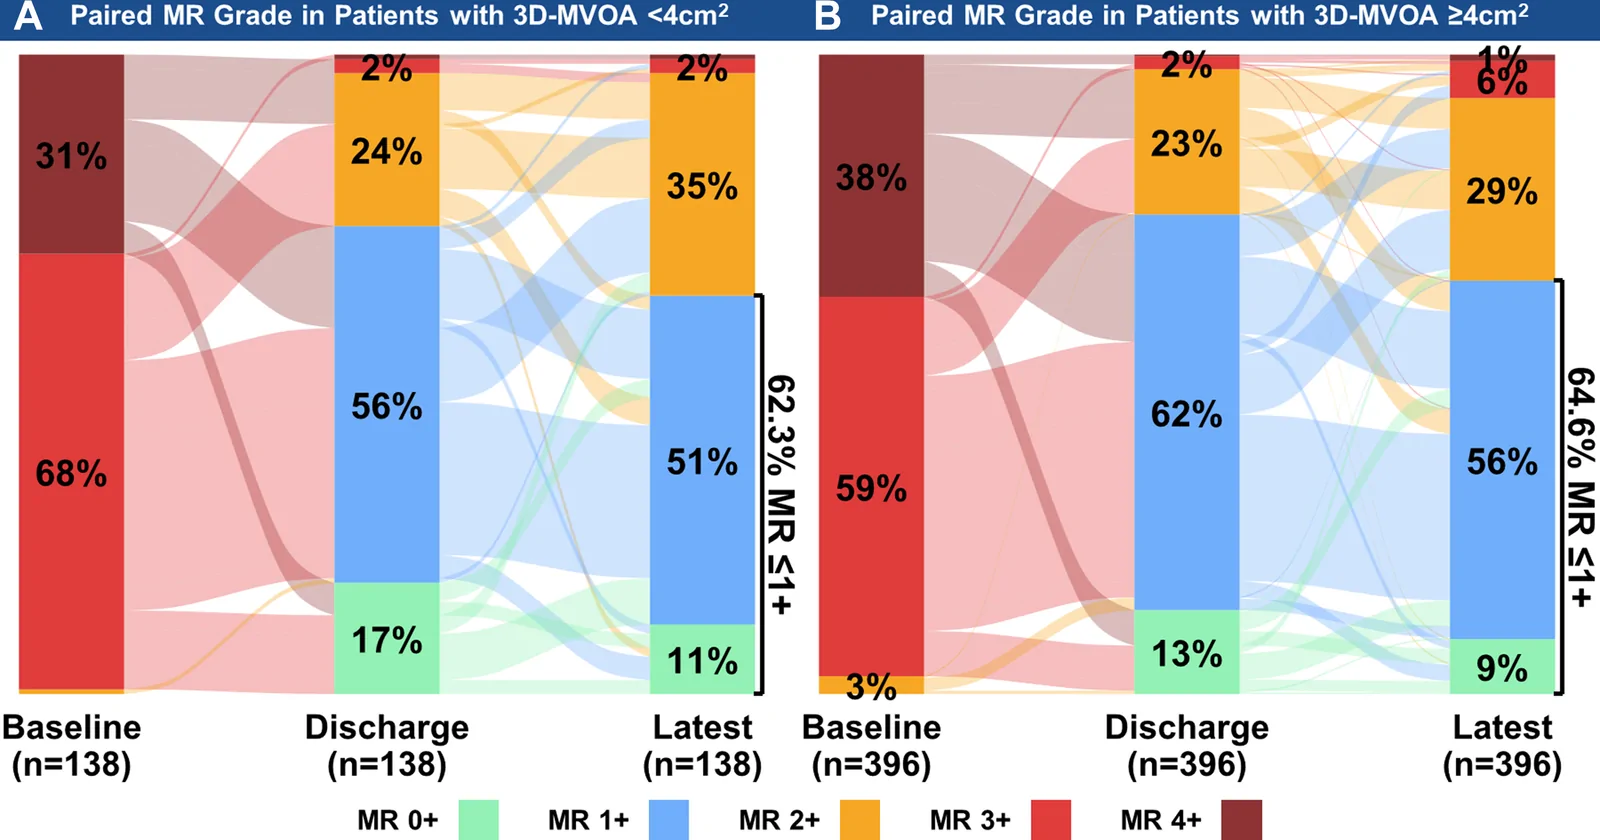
Paired MR Grade from Baseline to Latest Follow-Up Paired MR grade is depicted for (A) patients with 3D-MVOA <4 cm^2^ and (B) ≥4 cm^2^ with available echocardiographic assessments at baseline, discharge, and latest follow-up. Values < 1% are not displayed in the figure. In patients with 3D-MVOA <4 cm^2^ (A), 1 patient (0.7%, 1/138) had MR 2+ at baseline, 1 patient (0.7%, 1/138) had MR 4+ at discharge, and one patient (0.7%, 1/138) had MR 4+ at latest available echocardiographic follow-up. In patients with 3D-MVOA ≥4 cm^2^ (B), 1 patient (0.3%, 1/396) had MR 4+ at discharge. No difference was observed in the rate of MR ≤ 1+ at latest available echocardiographic follow-up (*P* = 0.698). 3D = three-dimensional; MR = mitral regurgitation; MVOA = mitral valve orifice area.
